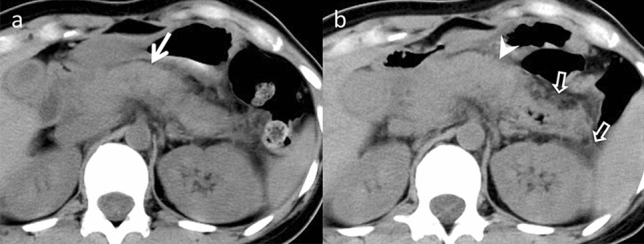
Drug-induced pancreatitis in a 30-year-old woman with abdominal pain, who was undergoing L-asparaginase therapy for acute lymphocytic leukemia. a Unenhanced computed tomography (CT) demonstrates the normal pancreas size before L-asparaginase therapy (arrow). b Pancreatic enlargement (arrowhead) and retroperitoneal fat stranding (open arrows) are observed on unenhanced CT performed one week after the L-asparaginase initiation. On imaging, acute pancreatitis from other causes is a differential; however, both are nonspecific and difficult to distinguish on imaging alone
